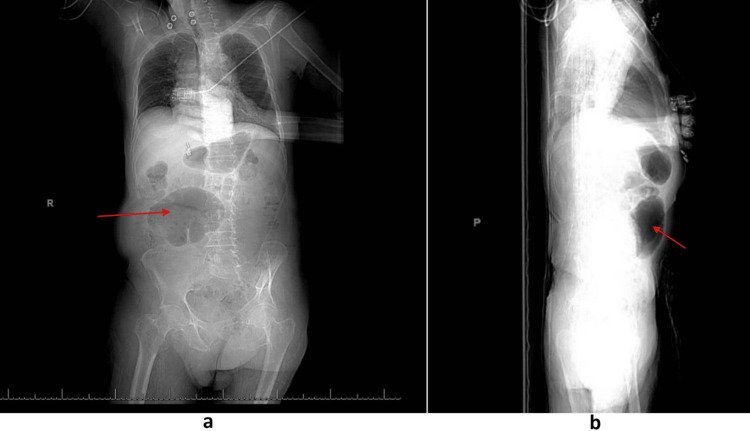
X-ray of the abdomen showing a hugely dilated loop of bowel with haustra filled with gas slightly to the right of the midline a) anterior-posterior view; b) lateral view
